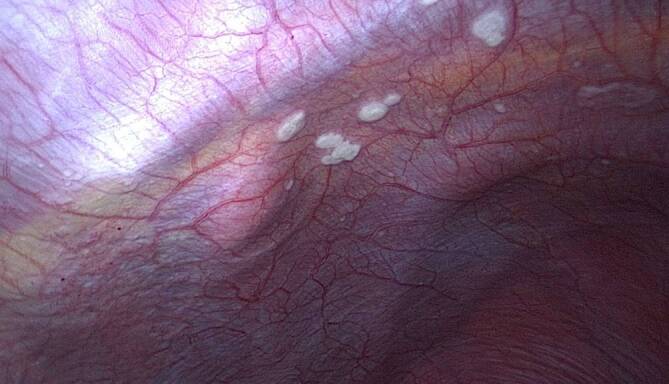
In der postoperativ angefertigten Computertomographie (CT) von Thorax und Abdomen (mit i.v. Kontrastmittel) zeigten sich hypodense Läsionen der Leber in Segment 6, 7 und 8, zystische Läsionen in Segment 4a sowie peritoneale Auflagerungen perihepatisch (Abb. 2). Weitere abdominelle oder pulmonale Manifestationen wurden ausgeschlossen. Die weiterführende Diagnostik inklusive Ösophagogastroduodenoskopie, Koloskopie und gynäkologischer Vorstellung ergaben keinen Anhalt für weitere Pathologien.
